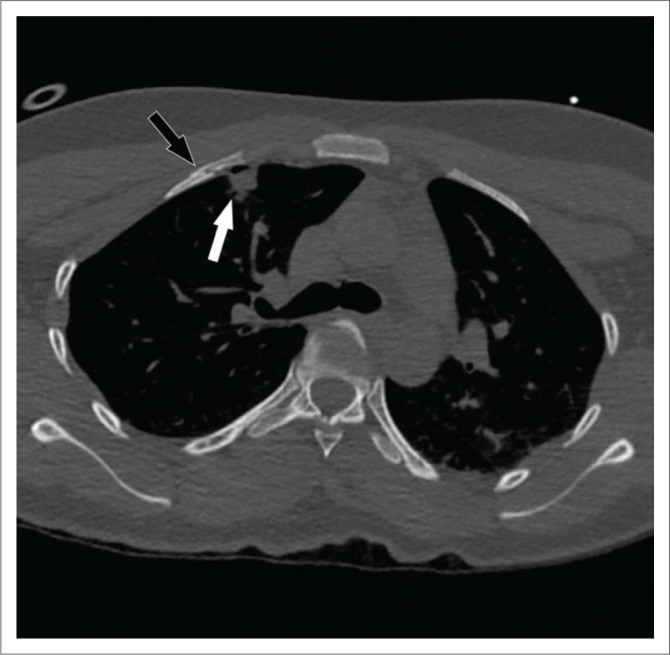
Axial bone reconstruction CT of a the chest demonstrating a minimally displaced second rib fracture (black arrow) associated with a small lung laceration and contusion (white arrow). As with cases 5 and 6, lung injuries such as contusions or lacerations should prompt careful search for associated rib fractures in the trauma patient.
